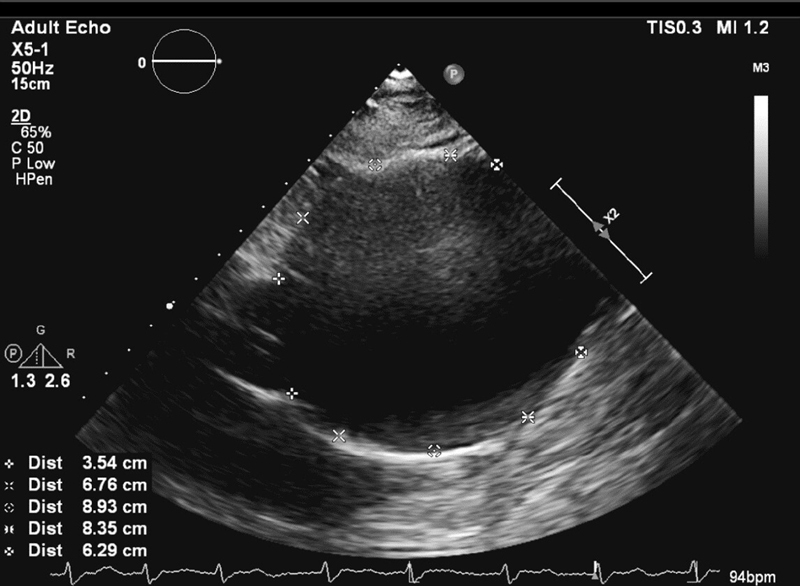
Transthoracic echocardiography showing a giant aortic aneurysm.
